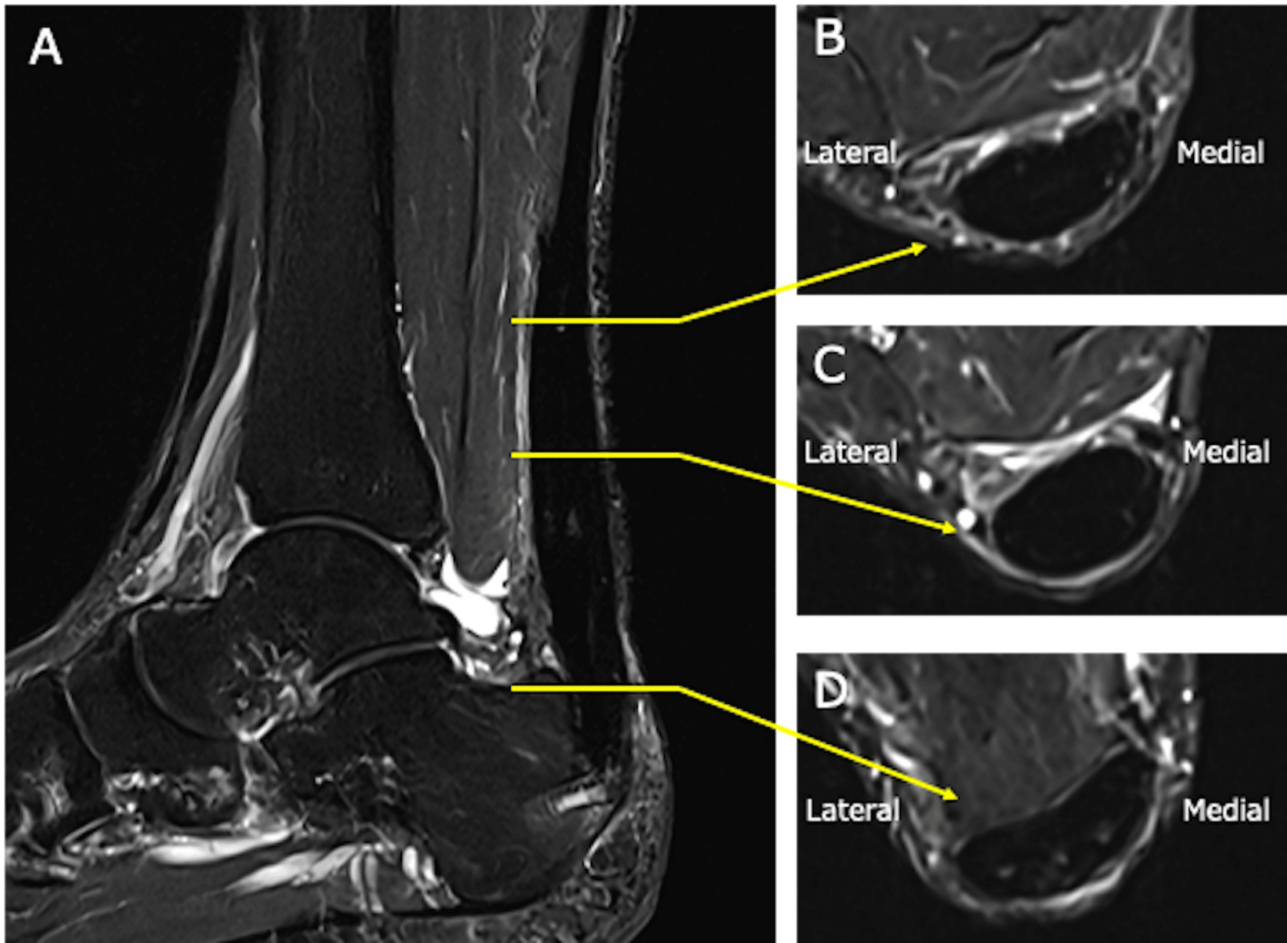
Magnetic resonance imaging at one year postoperatively Favorable healing of the Achilles tendon was observed. A: sagittal view of the Achilles tendon; B: axial view of the proximal Achilles tendon; C: axial view of the mid portion of the Achilles tendon; D: axial view of the proximal Achilles tendon
